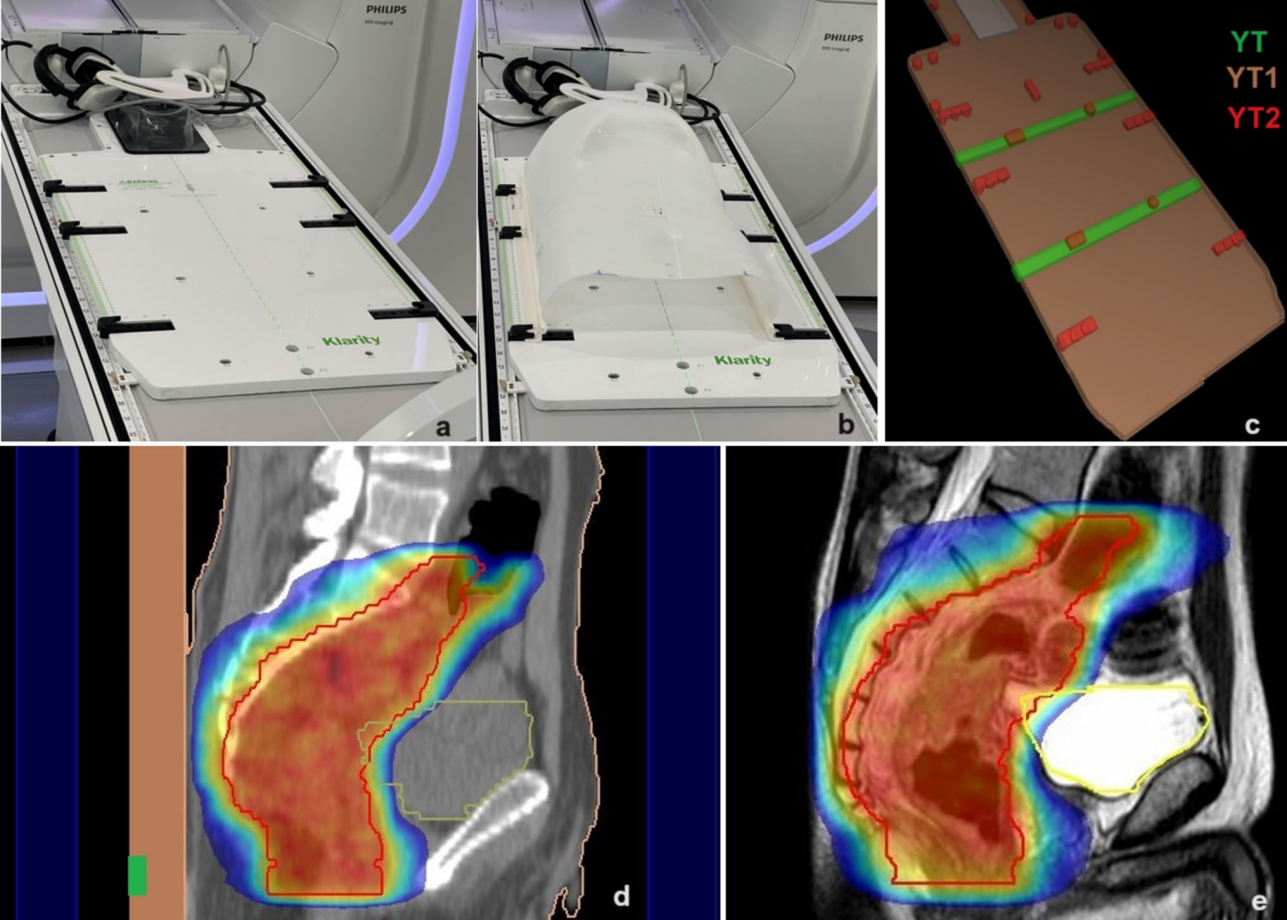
Patient positioning system a, b and its components YT, YT1, and YT2 in a 3D view c. ppd visibility d and non-visibility e on representative sagittal CT, and MRI images, respectively
